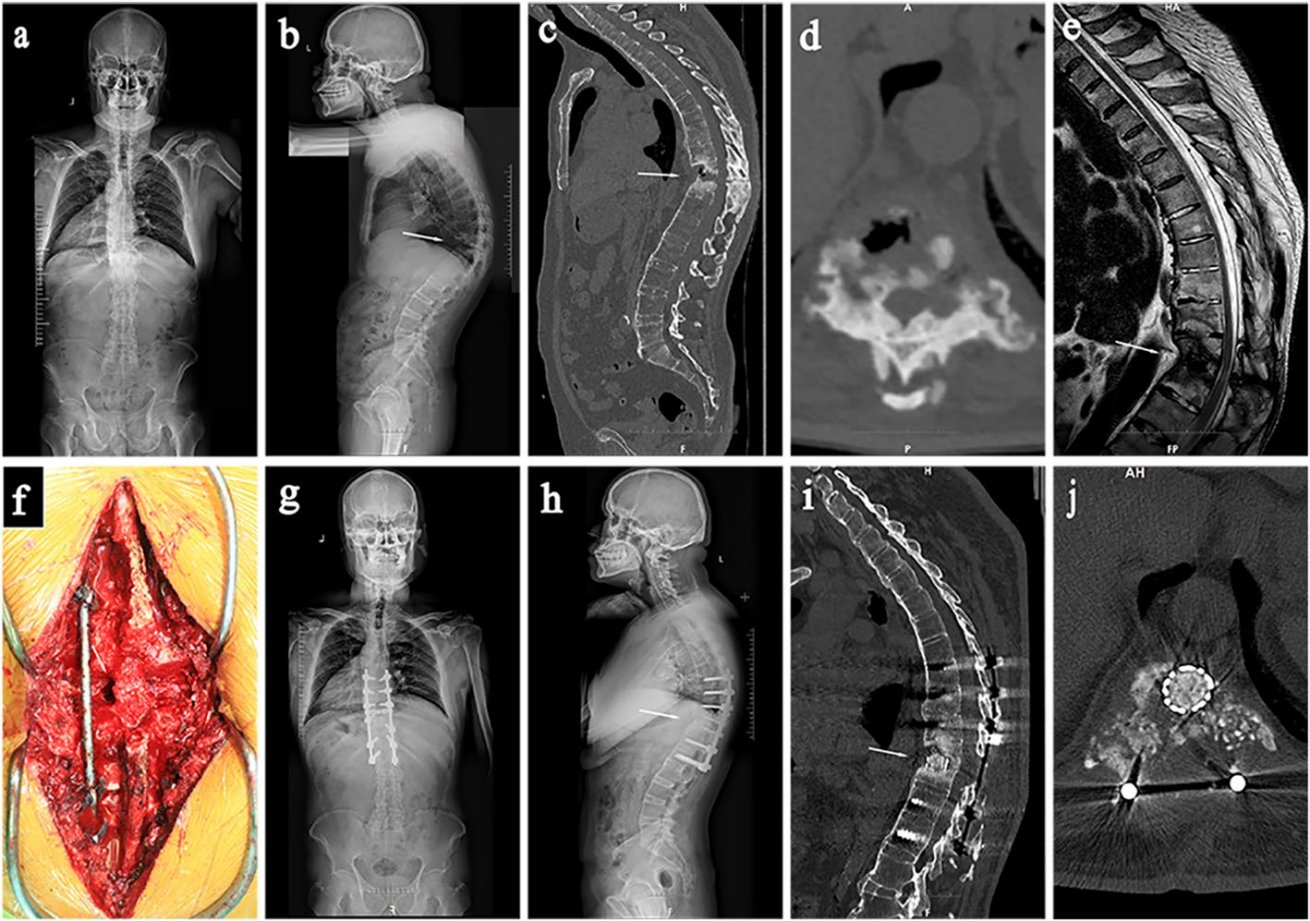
A 52-year-old male patient, diagnosed with AS combined with T10/11 AL. a Preoperative full length anteroposterior radiograph of spine. b Preoperative full length lateral radiograph of spine showed excessive thoracic kyphosis and a T10/11 intervertebral fracture line. c, d Preoperative CT sagittal view and cross section showed T10/11 intervertebral space involving a three-column fracture and a visible vacuum sign. e Preoperative MRI showed a low signal on T10/11 intervertebral space T2WI; f The osteotomy gap exposed through the fracture line during the operation. g Full length anteroposterior radiographs of the spine 18 months after surgery. h Full-length lateral spinal radiographs 18 months after surgery showed normal thoracic kyphosis, and a titanium cage was inserted into the T10/11 intervertebral space. i, j Sagittal and cross - sectional CT images at 18 months postoperatively showed intervertebral fusion with the bone graft
